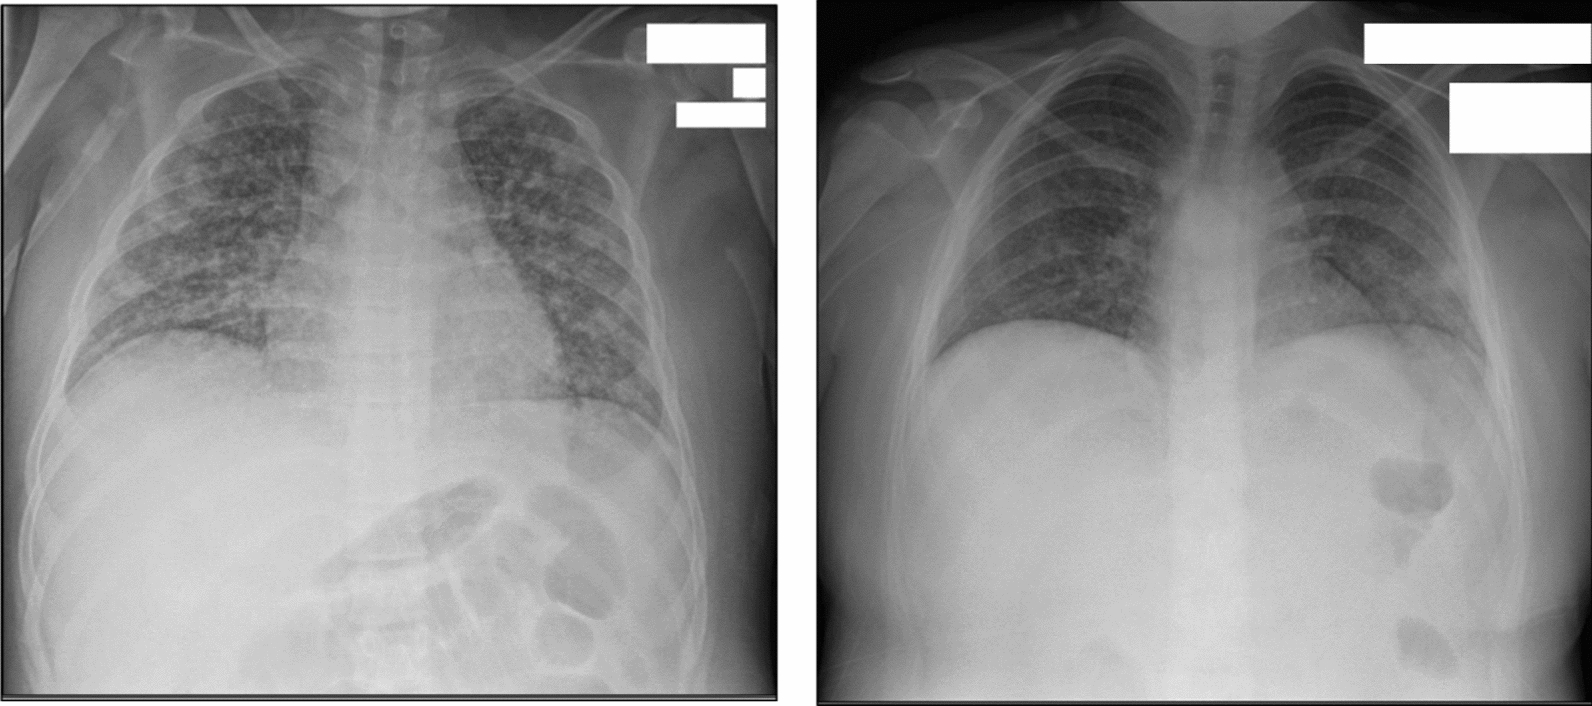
Initial chest radiograph of the patient on admission (left) showing diffuse bilateral heterogeneous infiltrates. After completion of intravenous liposomal amphotericin B and transition to posaconazole, the patient’s respiratory status improved with repeat chest radiograph (right) showing marked reduction in infiltrative pulmonary disease
